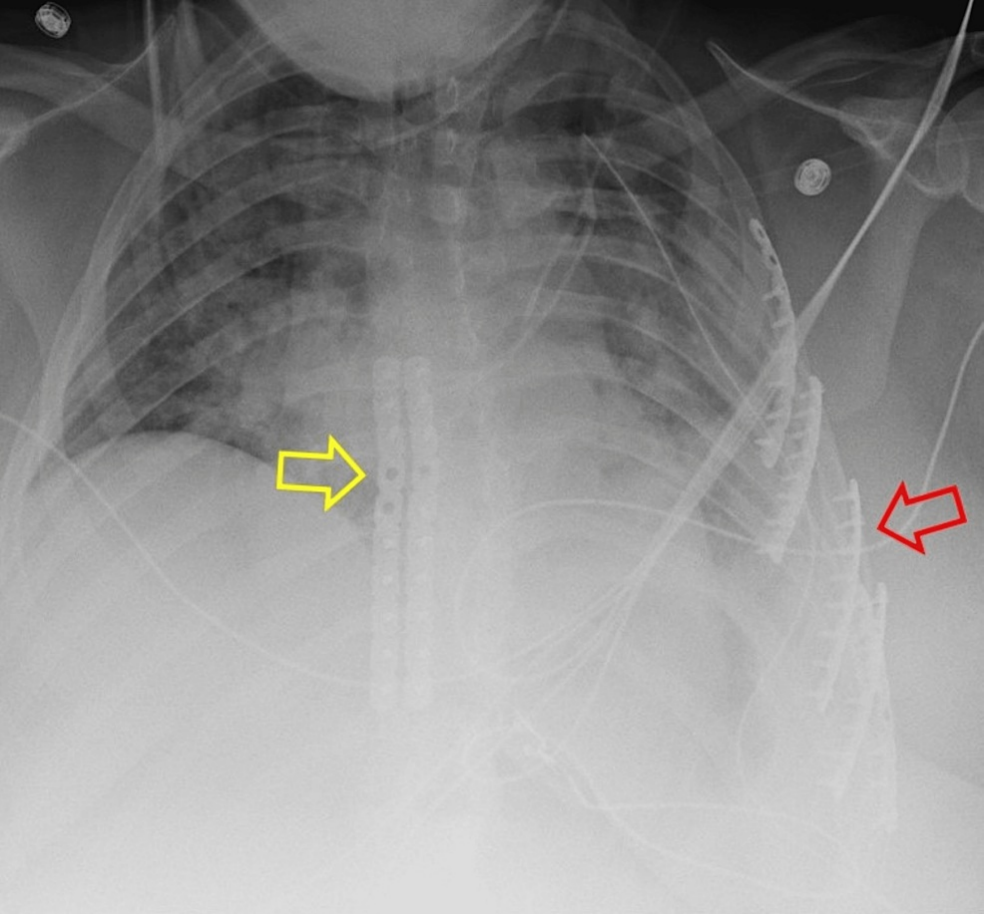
Post-operative chest x-ray. The yellow arrow indicates the sternal plates, and the red arrow indicates the rib plates.
